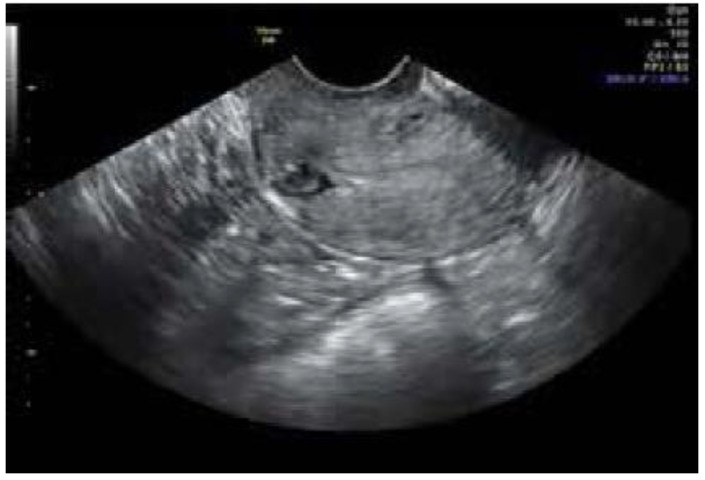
Transvaginal ultrasound can be used for in-office diagnosis. Ultrasound courtesy of Antony Dobson, MD, PhD.
